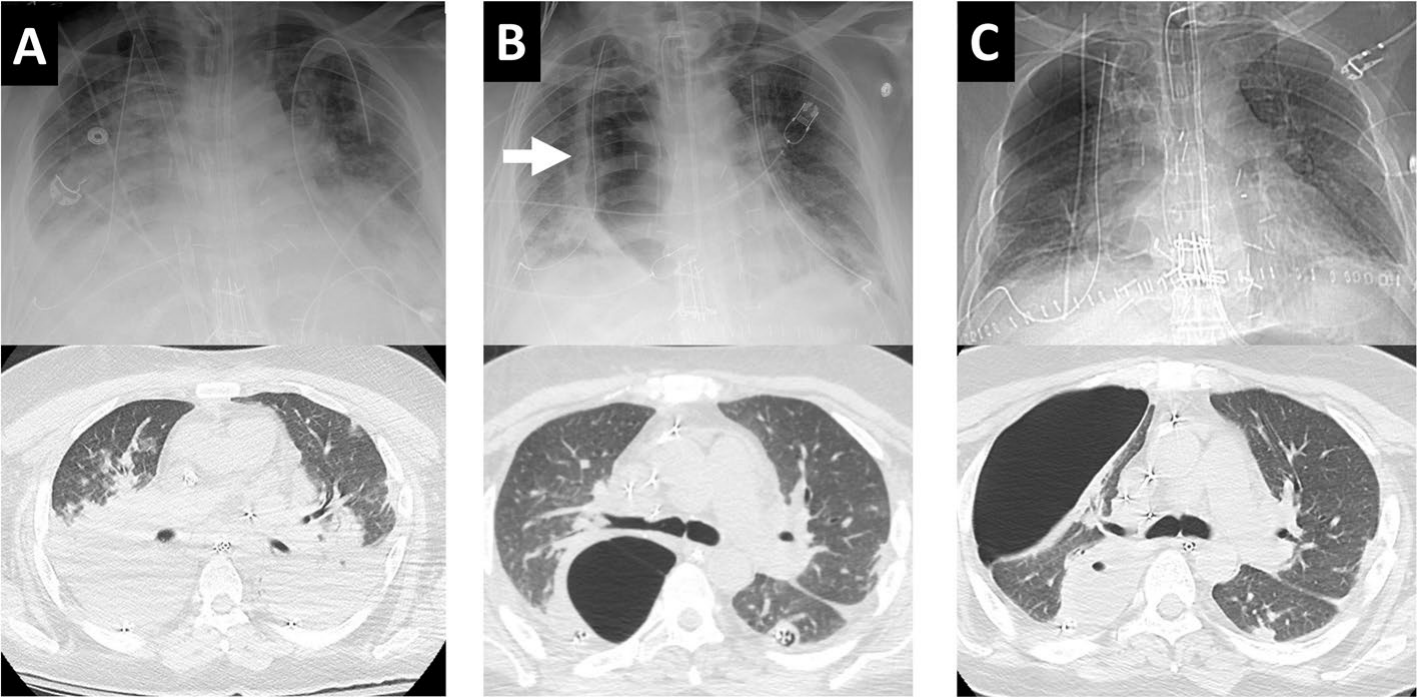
Chest roentgenogram and chest computed tomography. A Bilateral lower lobe pneumonia. B Pneumatocele. C Ruptured pneumatocele and pneumothorax. The arrow showed the pneumatocele
